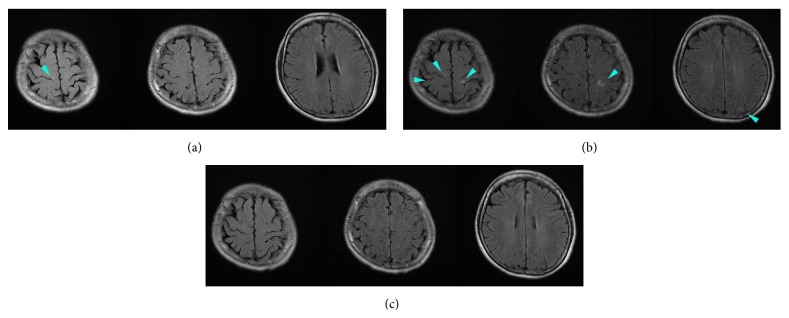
(a) Fluid attenuation inversion recovery (FLAIR) images of MRI on admission were interpreted as normal, but in retrospect, they show an indistinct high-intensity area in the right frontal subcortical white matter (arrowhead). (b) FLAIR images of MRI on the next day reveal high-intensity areas, presumed to be vasogenic edema, in the right frontal, bilateral parietal, and left occipital subcortical white matter (arrowheads). (c) The repeat MRI performed one month later shows the disappearance of all lesions.
